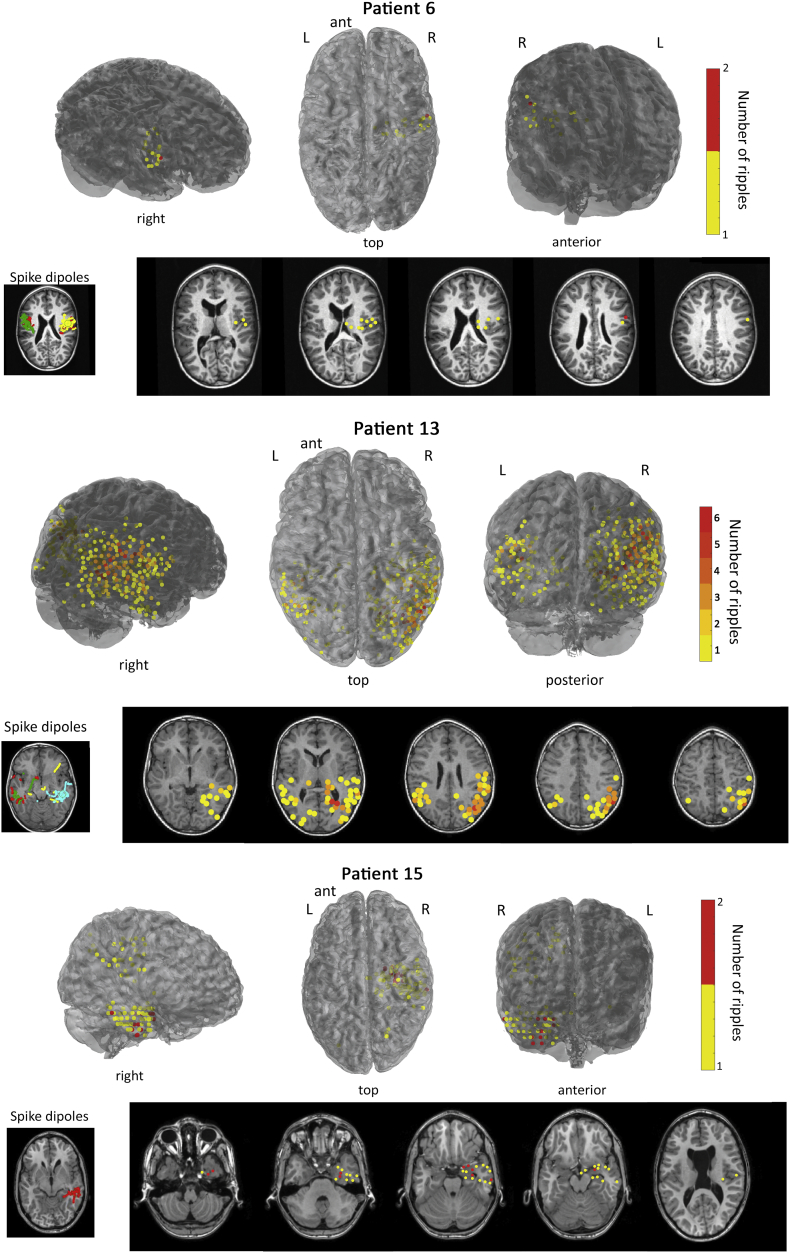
Ripple results for three patients. Ripples are visualized in a 3D figure (top), as well as axial MRI slices (bottom right). The ripple locations are compared to the spike dipoles from the clinical report (bottom left). All three patients show good concordance with MEG spike dipoles, as all ripple locations are also spike locations at lobar level. For patient 6, ripples were found unilaterally right centro-temporal, and spike dipoles were fitted bilateral centro-temporal. This was classified as good concordance, as the ripple location was also a spike location. Patient 6 did not undergo surgery because the number of seizures was too low. Patient 13 underwent surgery where a cortical tuber right frontal and a tuber right temporal were removed, but the seizure frequency did not change (Engel 4B). Patient 15 underwent a right temporo-lobectomy with amygdalohippocampectomy and was seizure free (Engel 1A). Postoperative MRI was not available.
